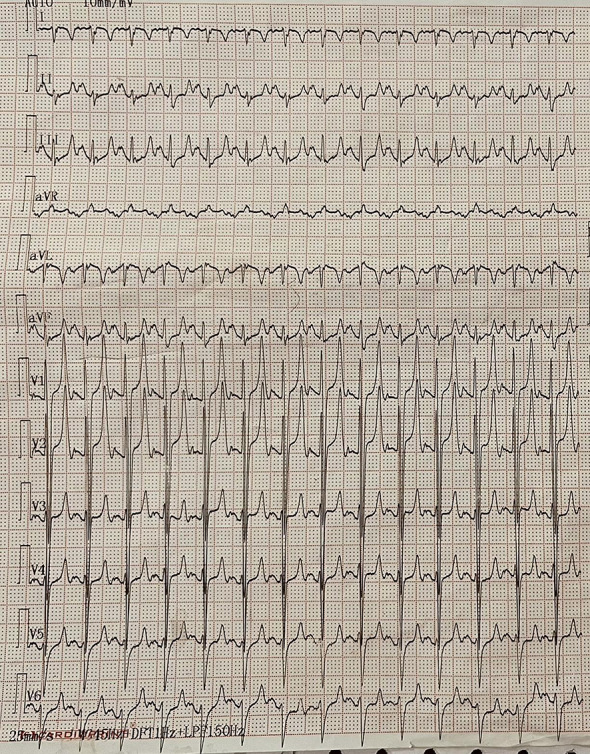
Digital chest x-ray revealed dextroversion, cardiomegaly possibly due to RVH, along with pulmonary vessel prominence to the right side and aortic prominence to the left side.
